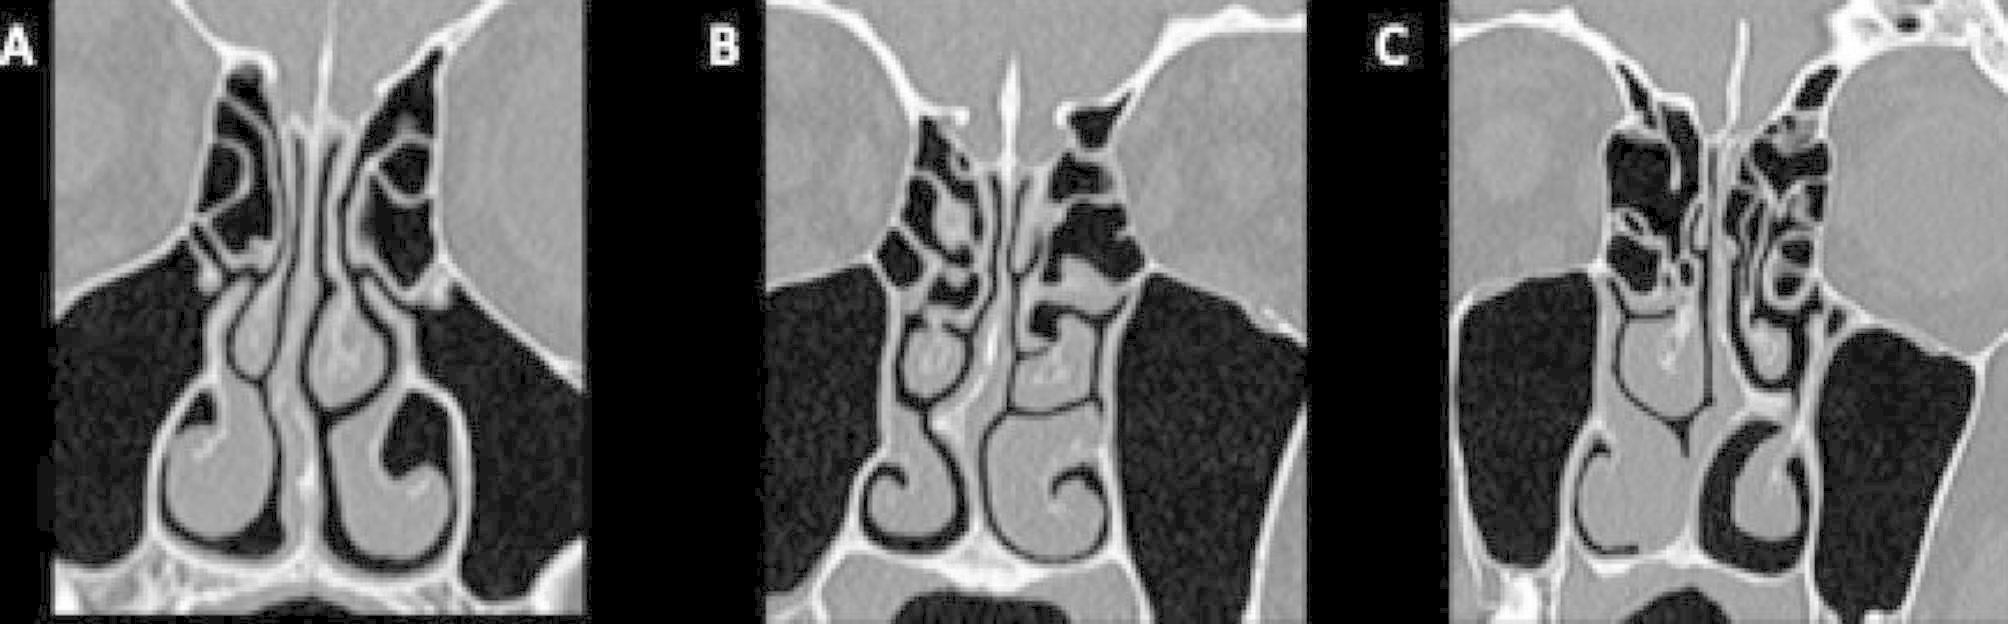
Dreher Classification of NSD on CT. Notes: A:Grade I, B:Grade II, C: Grade 3
